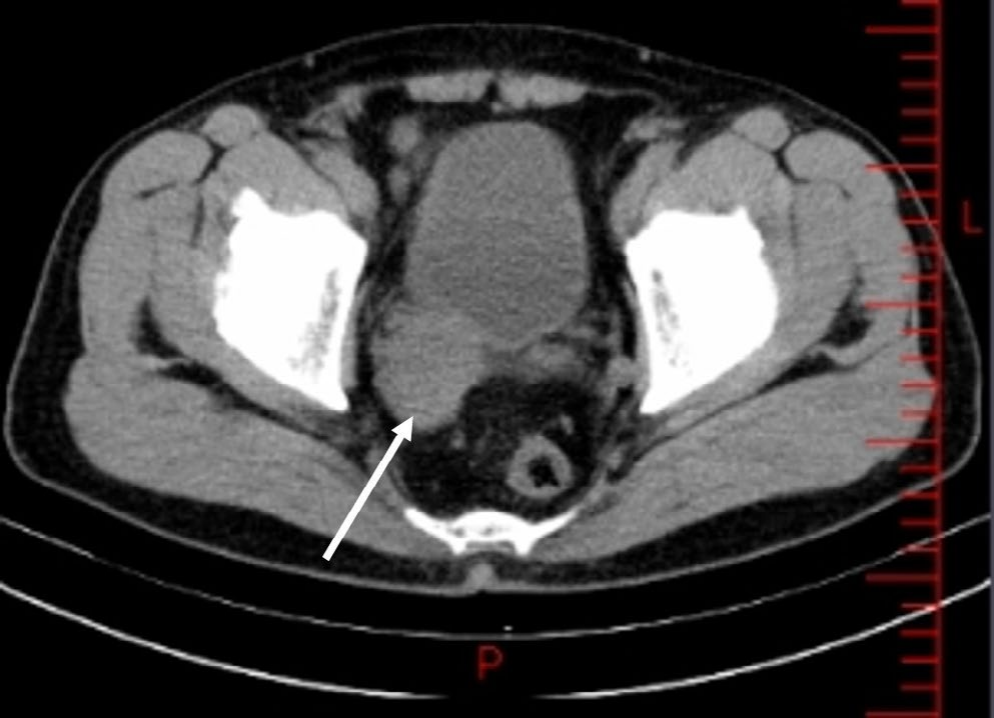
Axial CT scan showing a 44 × 35 mm mass in the right seminal vesicle causing ureteral compression.
